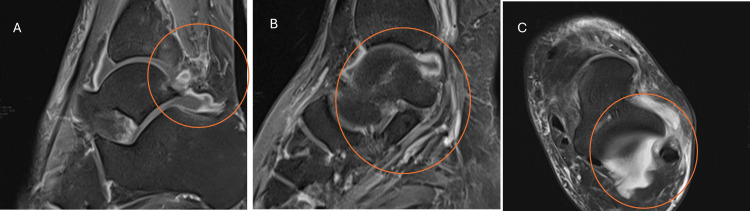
MRI of the left ankle indicating septic arthritis The magnetic resonance imaging (MRI) of the left ankle reveals a thick, peripherally enhancing effusion involving the talocrural and subtalar joints, with diffuse subcutaneous edema overlying the lateral malleolus. Panel A: Sagittal MRI of the ankle joint. This view shows the (hyperintense) signal in the joint cavity, suggesting joint effusion due to septic arthritis. Septic arthritis often causes an increase in synovial fluid, visible as bright areas on T2-weighted images. Panel B: Another sagittal view of the ankle with a closer focus on joint structures. Panel C: Axial MRI view of the ankle, providing a cross-sectional perspective. This view shows the distribution of effusion within the joint space and can reveal the spread of infection to the surrounding soft tissues.
